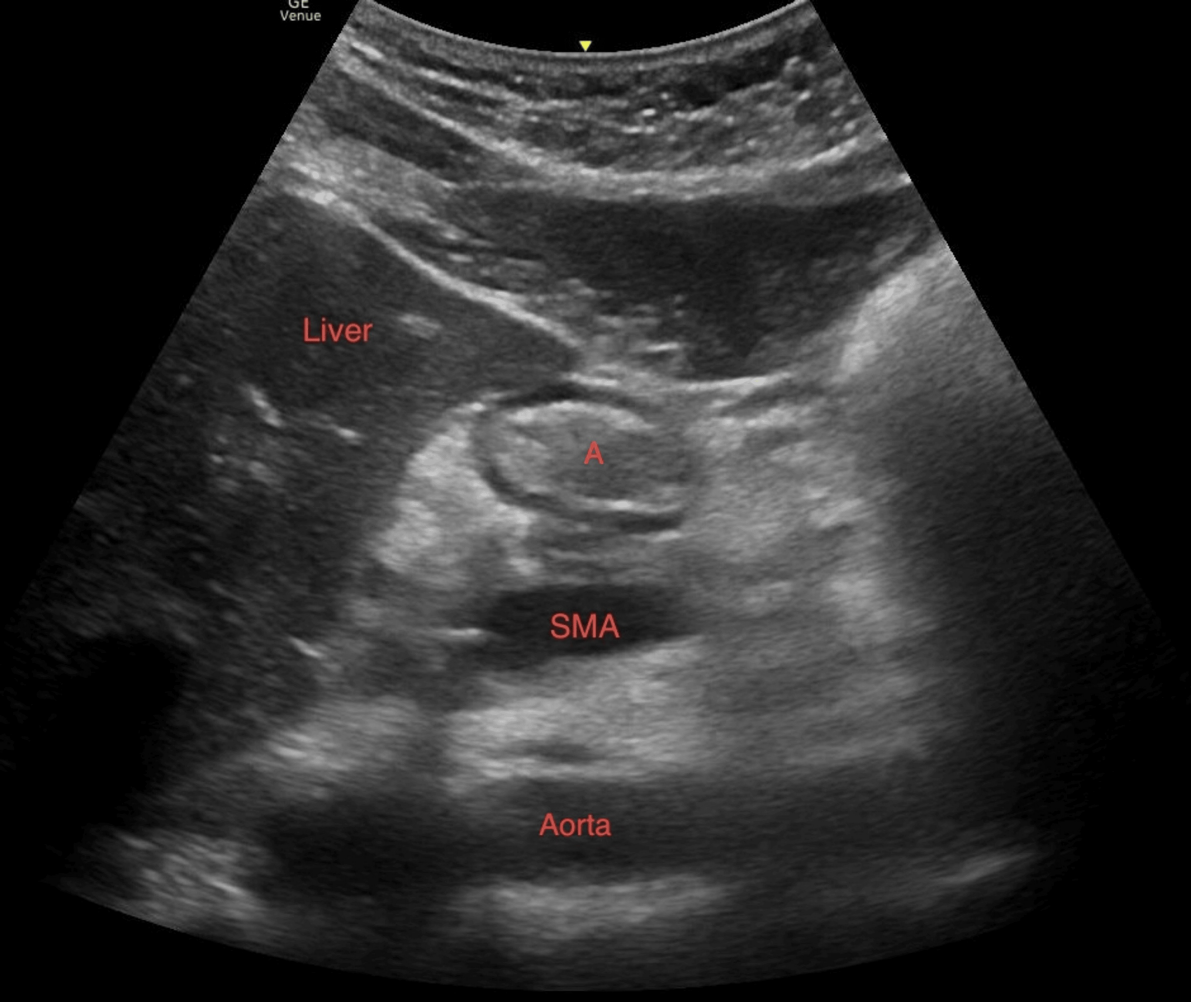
Sonographic image of the gastric antrum in the right lateral decubitus position A: antrum, SMA: superior mesenteric artery
